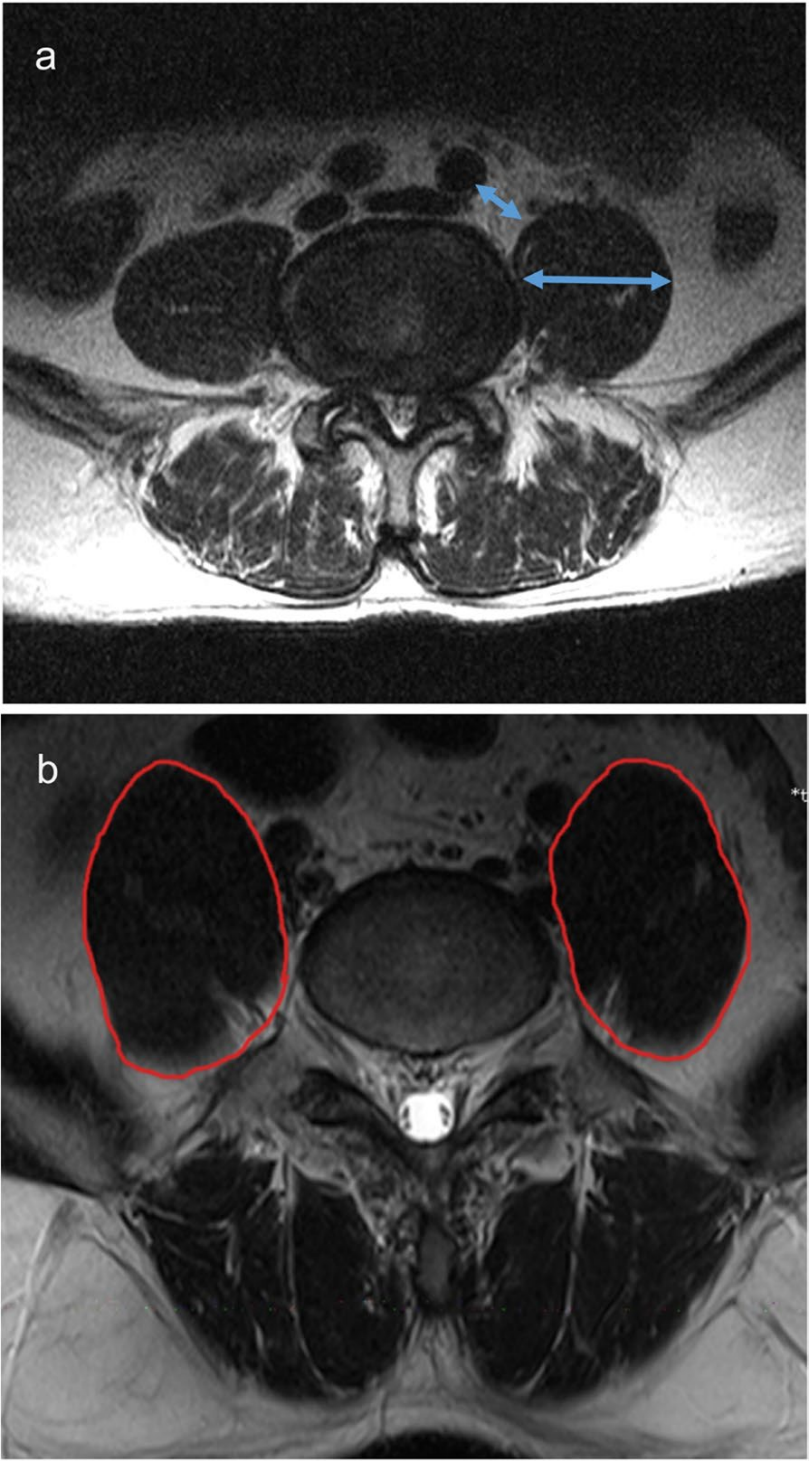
a Measurement of the size of the space between psoas major muscle and large artery, and the thickness of psoas major muscle by preoperative axial T2-weighted MRI image at surgical segment. b Typical psoas major muscle elevation from axial T2-weighted MRI images at surgical segment
